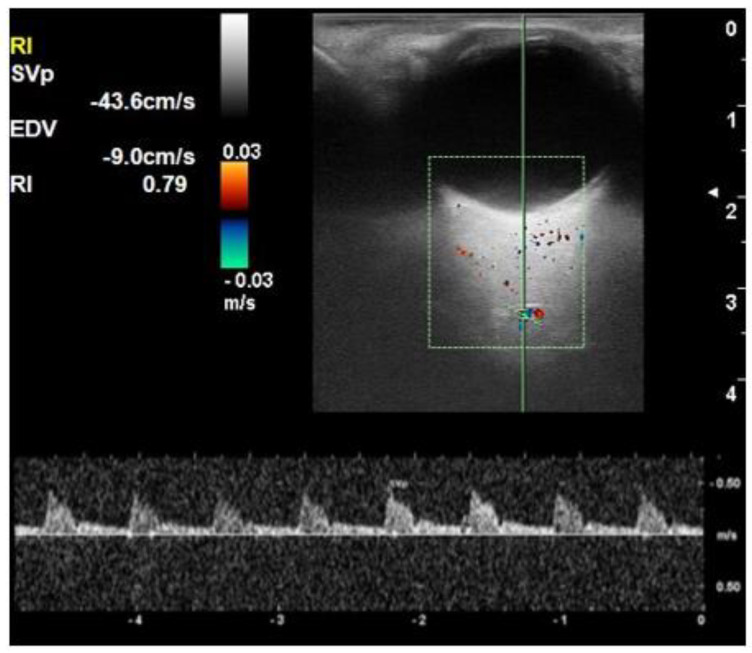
An orbital Doppler image in a patient with diabetic retinopathy shows a resistive index of 0.79.
